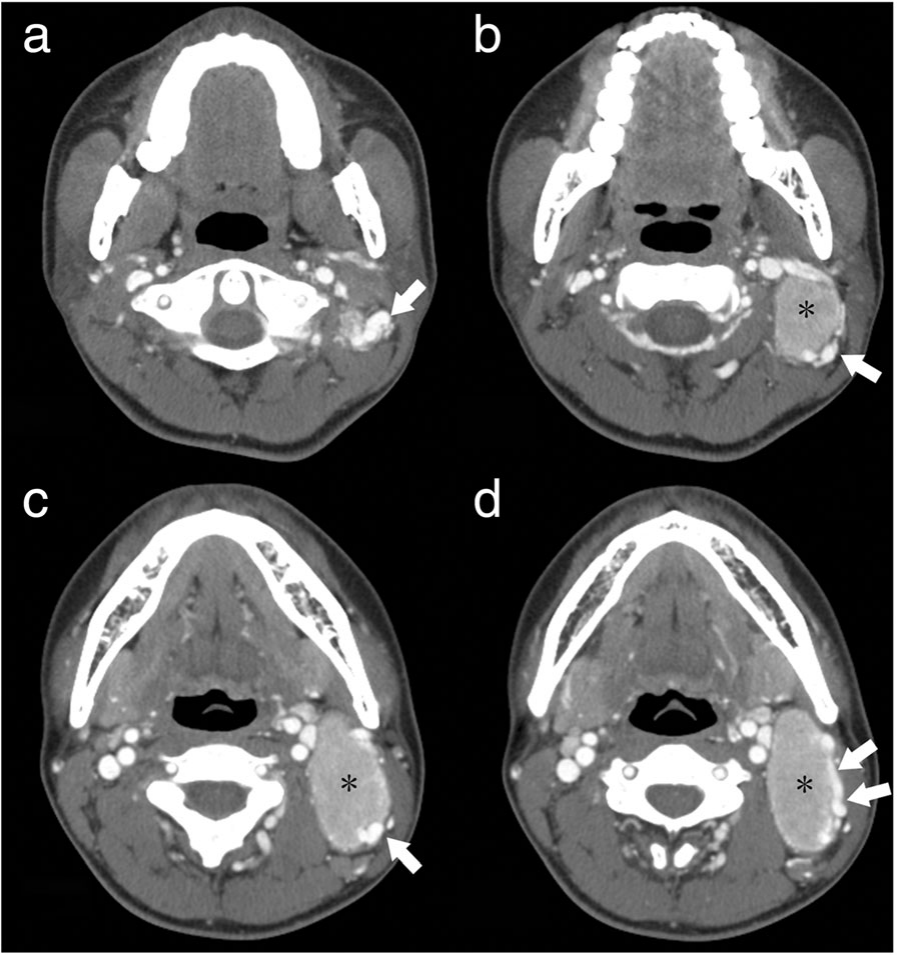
A 29-year-old man who presented with hyaline vascular type Castleman disease. a-d Contrast-enhanced CT images of the neck show a solitary nodule (black *) with a marked enhancement pattern and dilated and tortuous vessels in the periphery of the nodule (white arrows)
